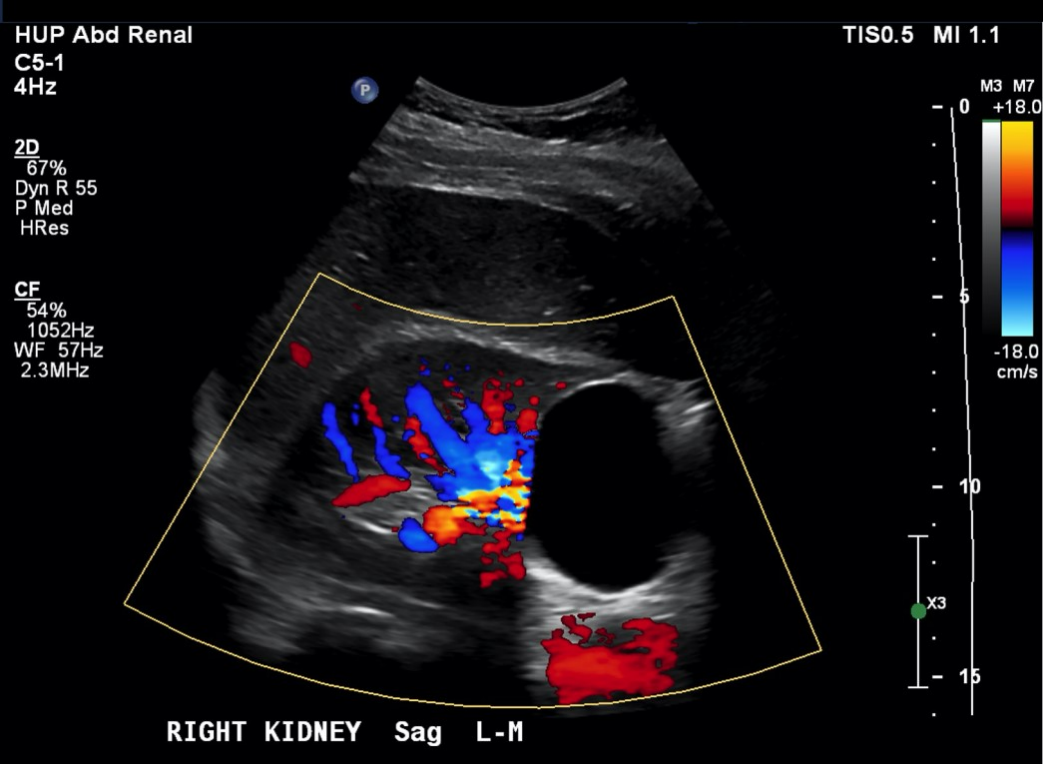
Doppler mode sagittal imaging plane of right kidney from case 1 demonstrating patent renal vasculature and absence of flow within the cyst.
